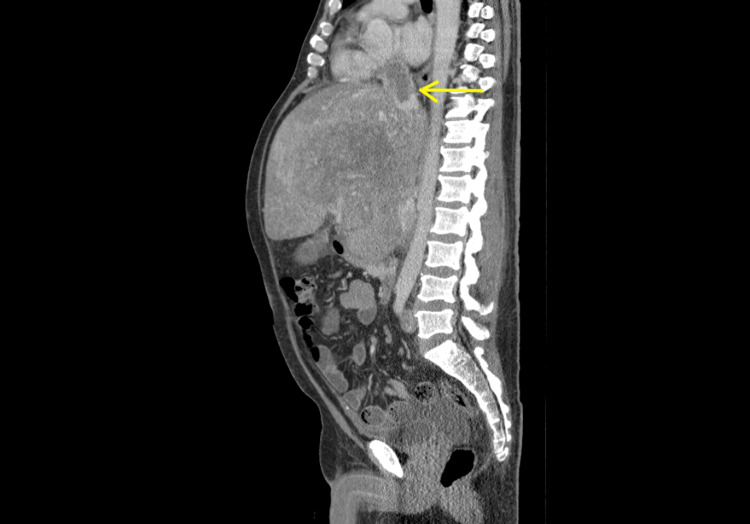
Sagittal view of the repeat CT of chest and abdomen with contrast Seen is the extension of the tumor to the IVC and the RA (yellow arrow). IVC: Inferior vena cava, RA: Right atrium
